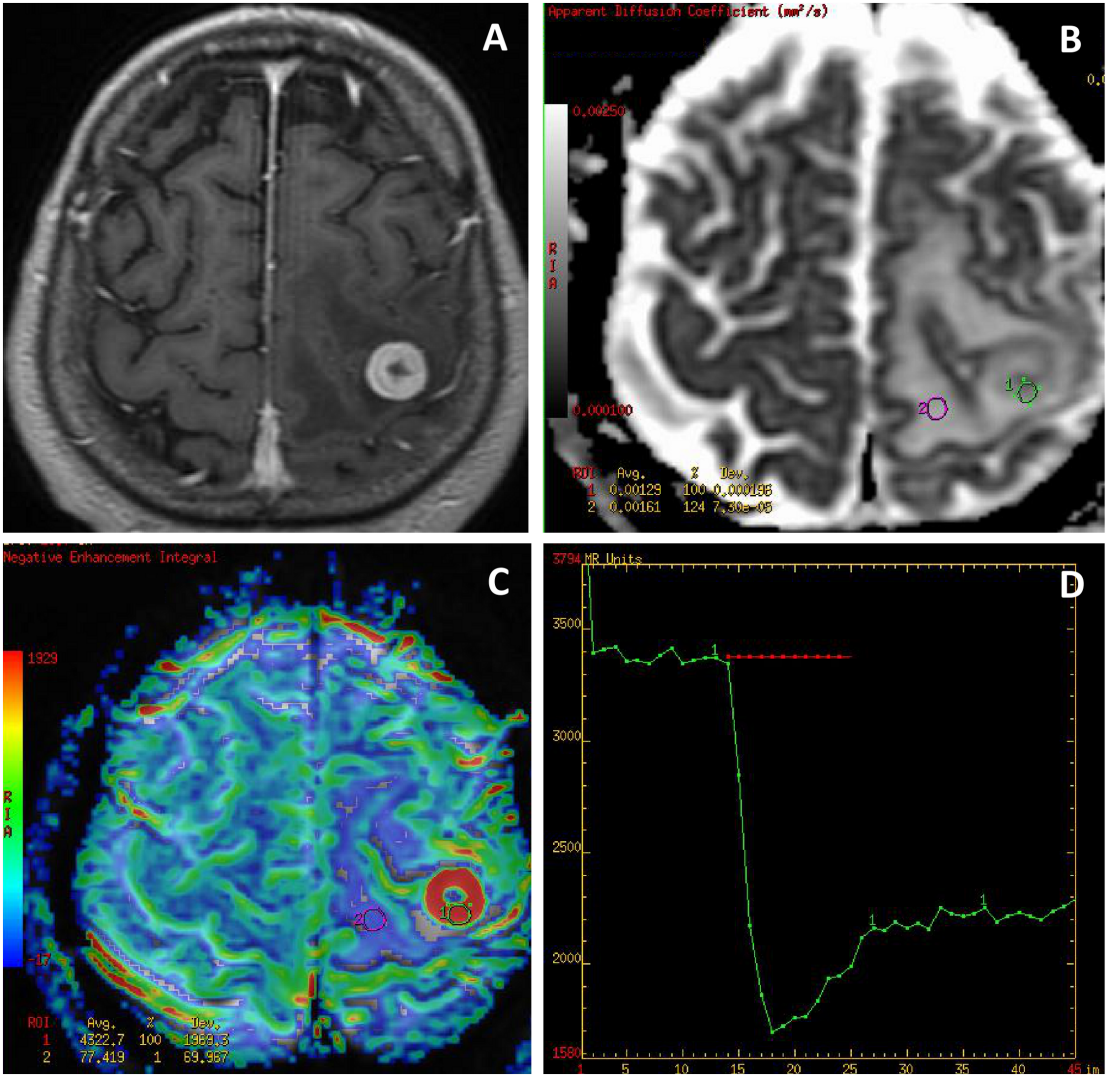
A solitary metastasis. (A) a post-contrast T1-weighted image, (B) an ADC map, (C) a CBV map, (D) a perfusion signal intensity time curve. The ADC map (B) shows facilitated diffusion in both the tumor core (min ADC = 1.0 x 10^−3^ mm^2^/s) and in the peritumoral zone (min ADC = 1.32 x 10^−3^ mm^2^/s). The CBV map (C) shows a highly perfused tumor (max rCBV = 17.9) and no hyperperfusion within the peritumoral zone (max rCBV = 0.78) typical for pure vasogenic edema. The perfusion curve (D) presents partial return to the baseline (red transverse line).
